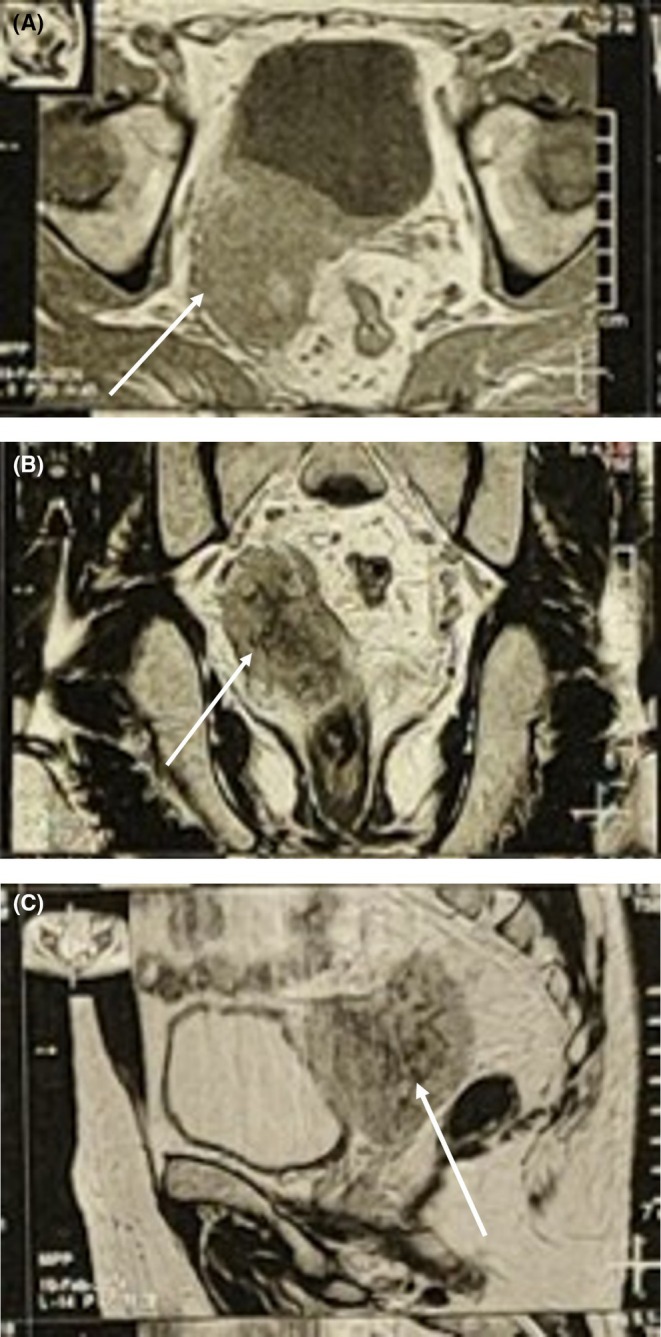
Biparametric MRI of the right seminal vesicle mass. (A) Axial T1‐weighted image showing a hyperintense mass with adjacent compression. (B) coronal T2‐weighted image demonstrating the mass with ureteral compression effects. (C) Sagittal T2‐weighted image highlighting the extent of the mass and its impact on surrounding structures.
